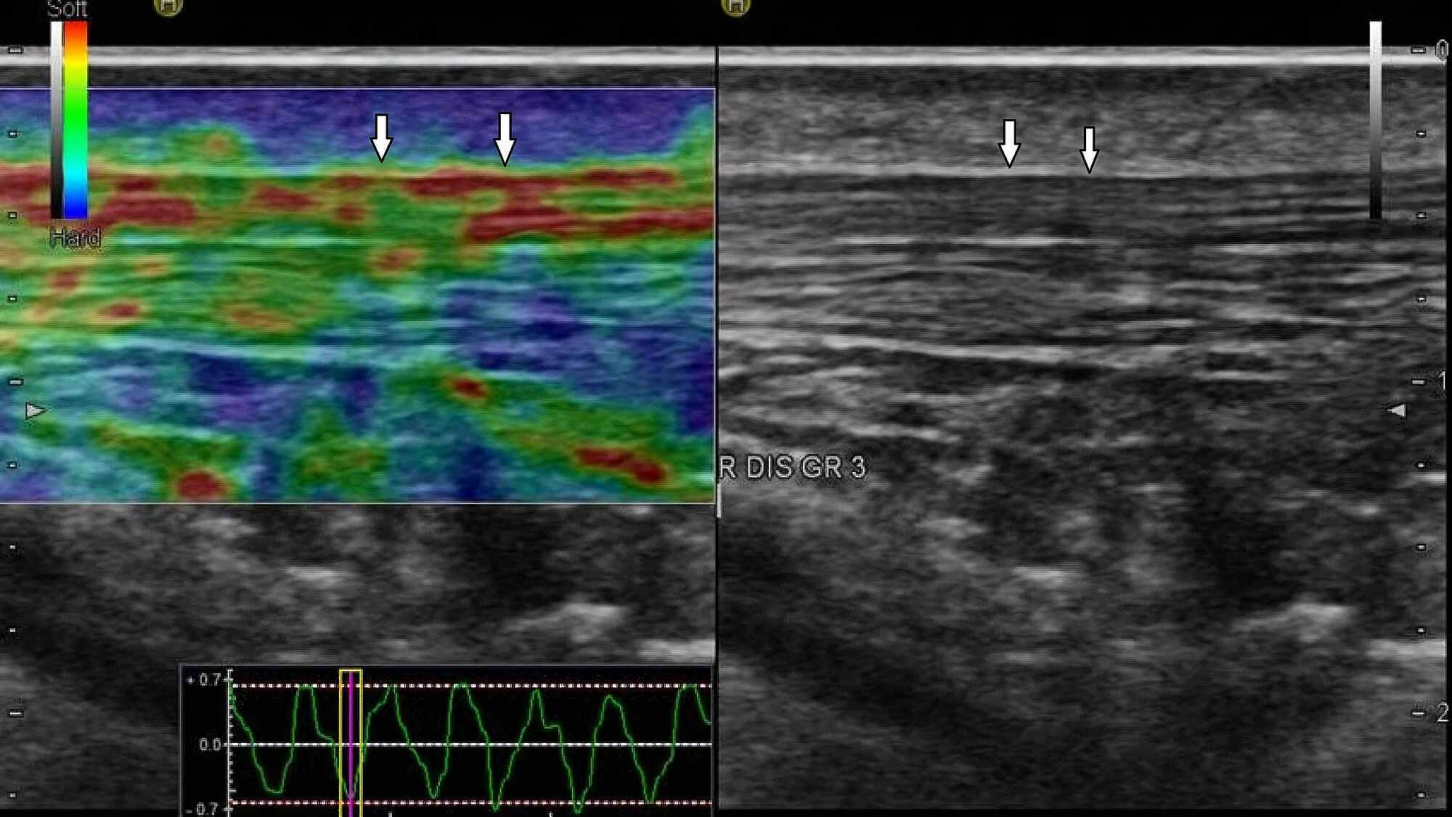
Grade 3 Achilles tendon. B-mode (right image) and sonoelastographic (left image) ultrasound images of the distal part of right Achilles tendon (arrows) in a 32-year-old woman with familial Mediterranean fever (FMF). Structurally impaired  tendon in shades of yellow to red.
